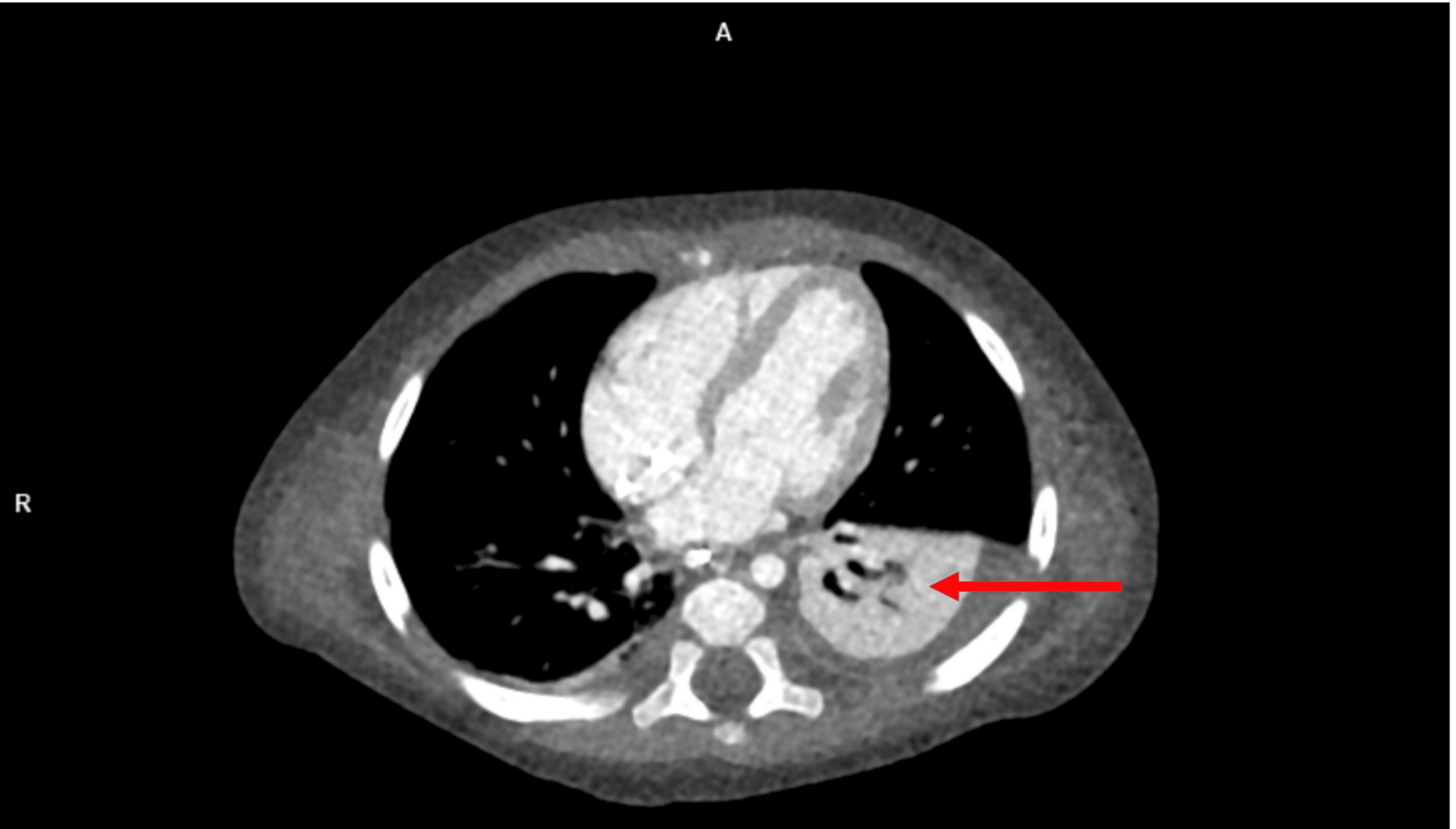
Non-contrast lung CT scan with a red arrow showing free fluid accumulation.
